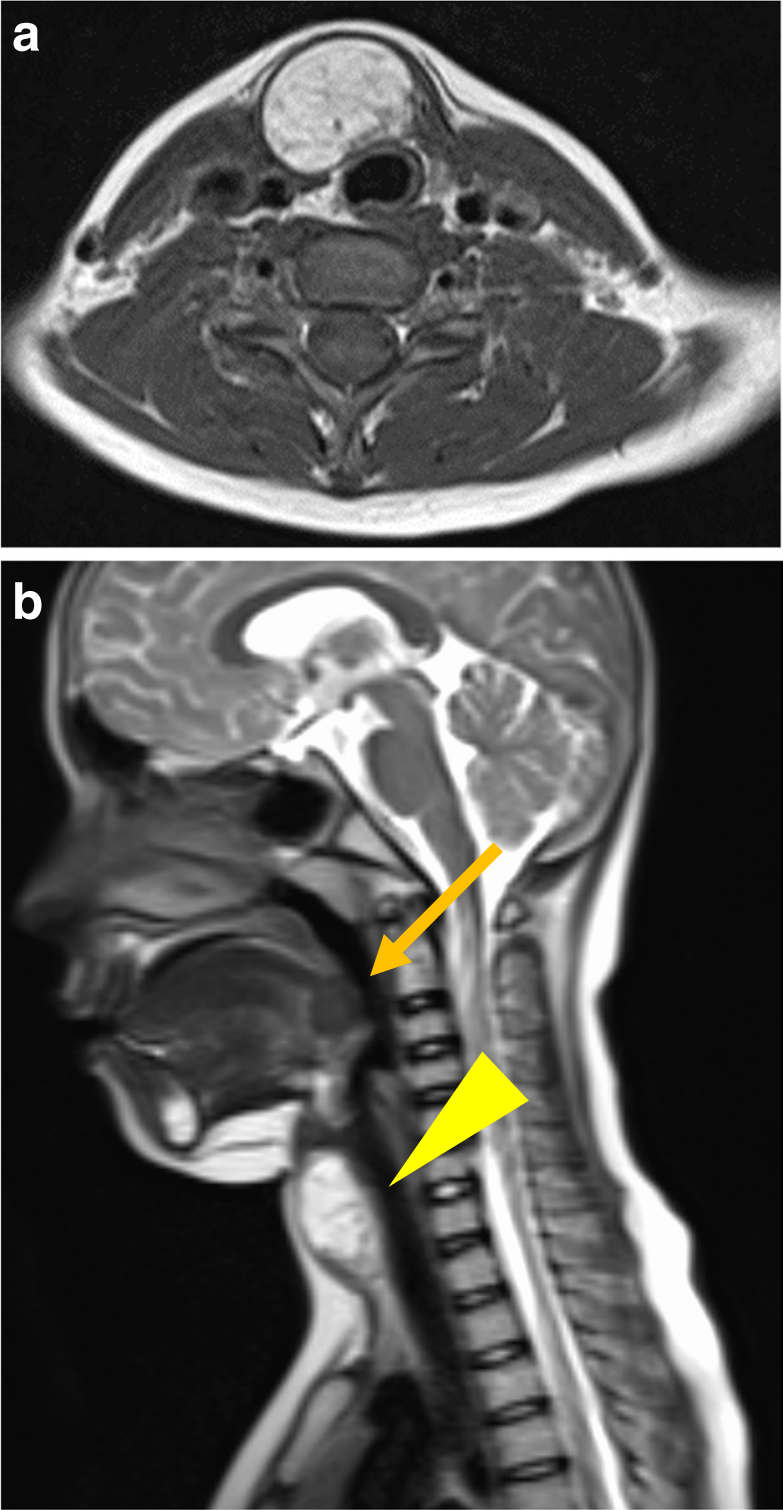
a, b MRI shows a high-intensity lesion on both T1- and T2-weighted images. The mass showed no suppression on the fat suppression images. The masses were seen in the sublingual lesion (arrow) and pretracheal region below the hyoid (arrow head)
